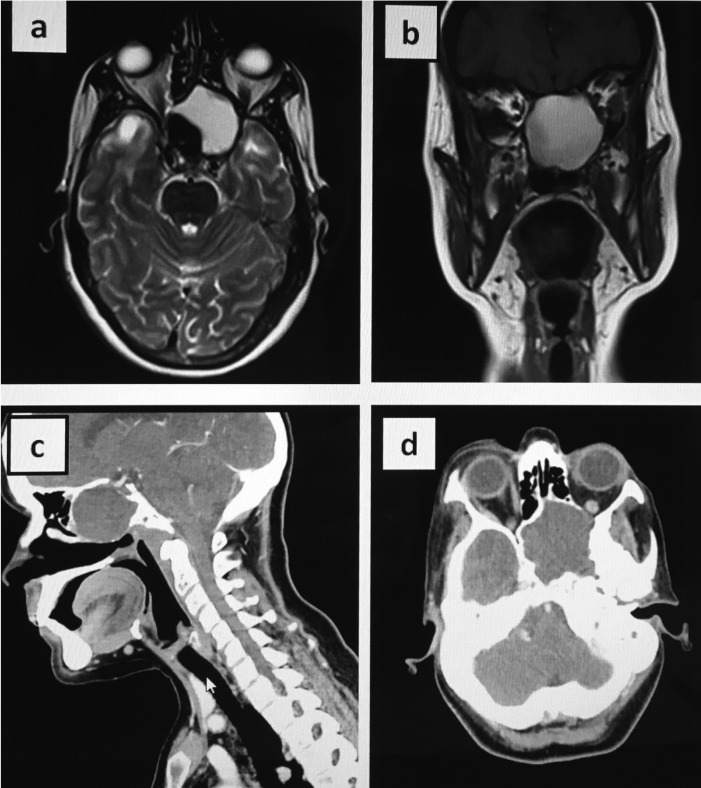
Radiological Images: Magnetic Ressonance (MR). Mucocele shows high signal intensity in both T1 and T2 weighted images. a: T2 axial MR, b: T1 coronal MR, c: sagital CT, d: axial CT.
